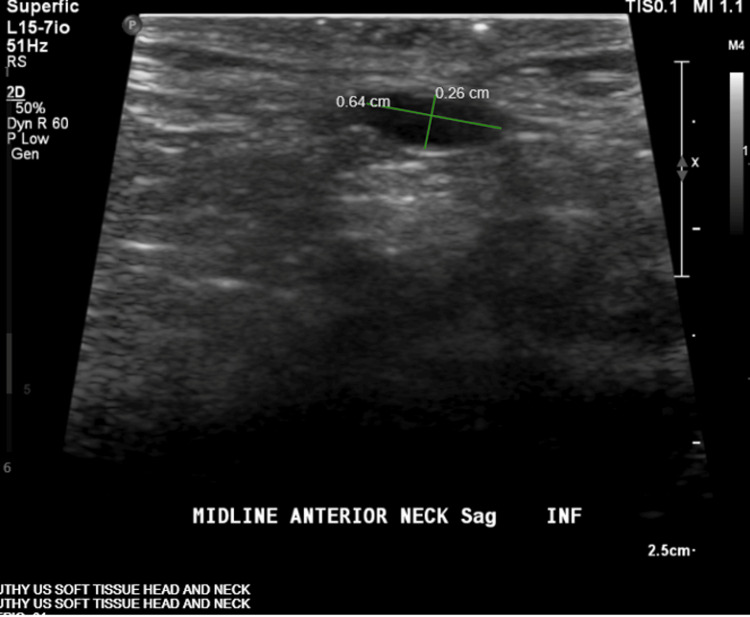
Ultrasound of the anterior neck demonstrating a 0.6 × 0.3 × 0.4 cm cystic lesion within the subcutaneous tissues at the level of the thyroid, with a 0.2 cm hypoechoic focus superiorly and an additional 0.2 cm hypoechoic/cystic area caudally, consistent with congenital midline cervical cleft.
